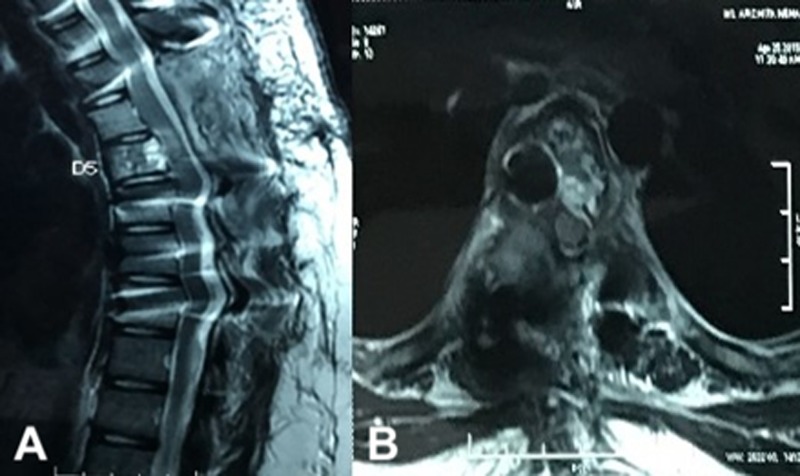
Post second intra-lesional resection MRI showing removal of epidural compressive mass. Dorsal spinal cord decompressed ventro-dorsally on sagittal (A) and axial views (B) No evidence of enhancing fluid filled lesion on T2WI of MRI.
